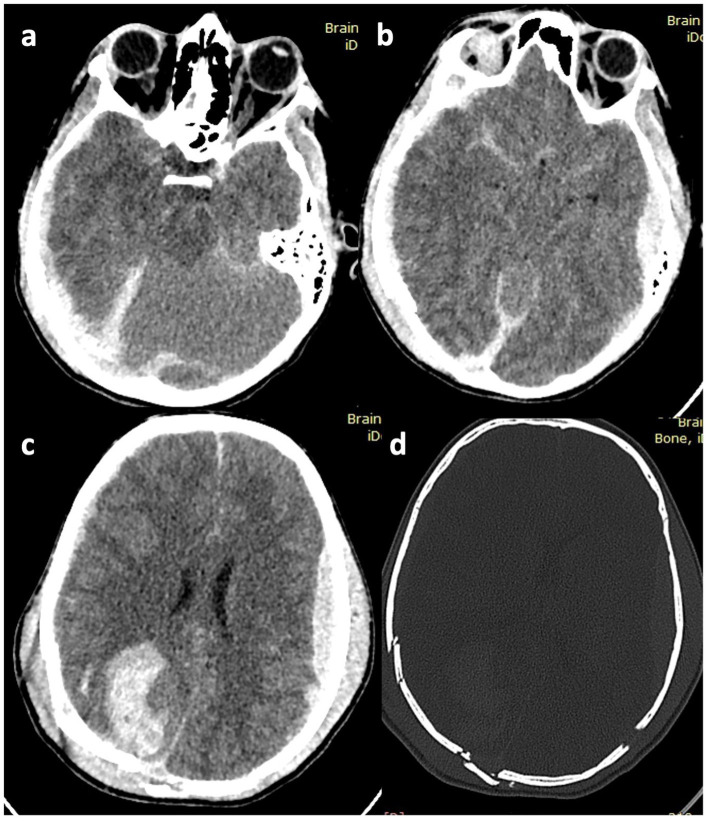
Head CT scan of a patient with severe traumatic brain injury showing: (a) diffuse brain edema, complete obliteration of the basal cisterns along with subarachnoid hemorrhage, (b) left acute subdural hematoma, (c) large right parietal intracerebral hematoma and adjacent cerebral contusions along with contralateral acute subdural hematoma, (d) multiple fractures of the cranial vault crossing the superior sagittal sinus on both sides.
